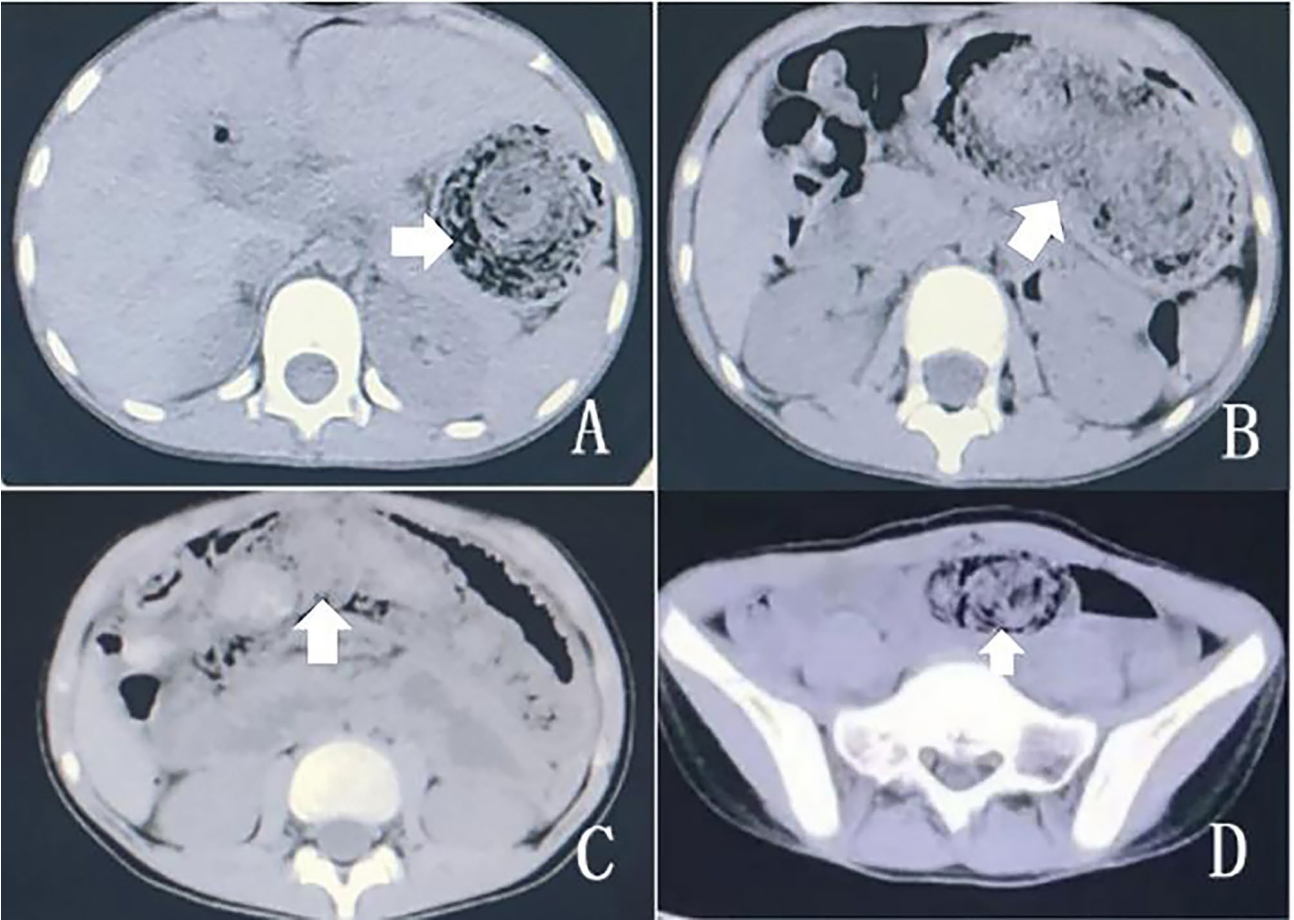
(A, B) Computed tomography scan of the abdomen showing intragastric BZs. (C, D) Small intestinal BZs.
